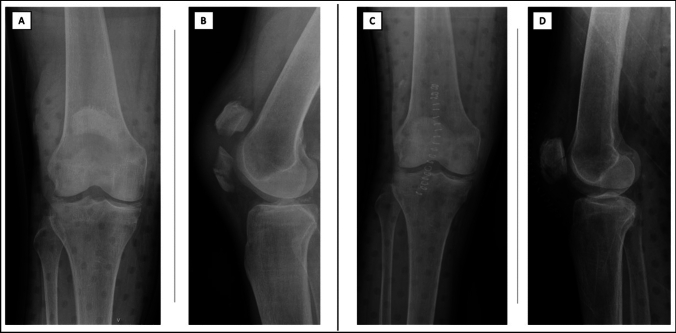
Pre- and postoperative imaging of patellar fractures treated with nonmetallic open reduction and internal fixation (ORIF). A preoperative anteroposterior X-ray and B preoperative lateral X-ray. C postoperative anteroposterior X-ray and D postoperative lateral X-ray
